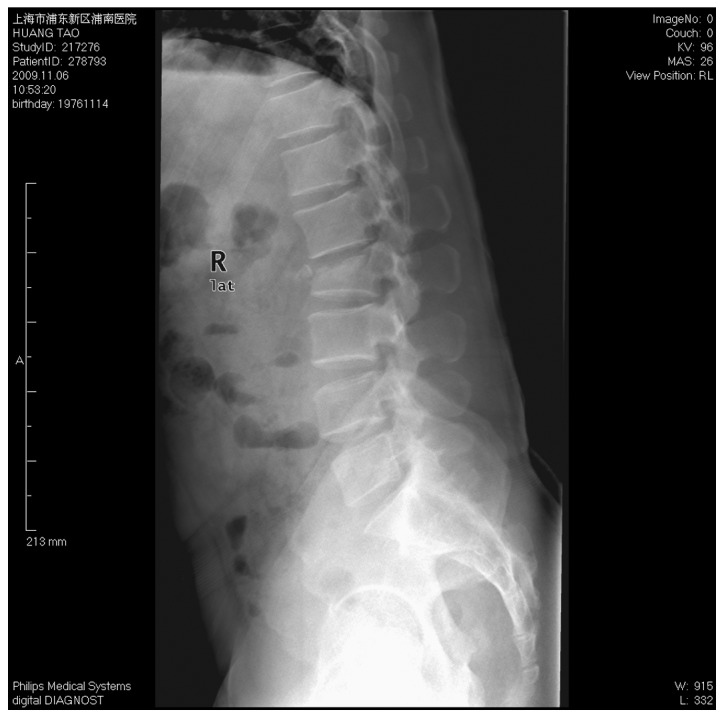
Lateral radiograph prior to triplane fixation of an L2 fracture.
